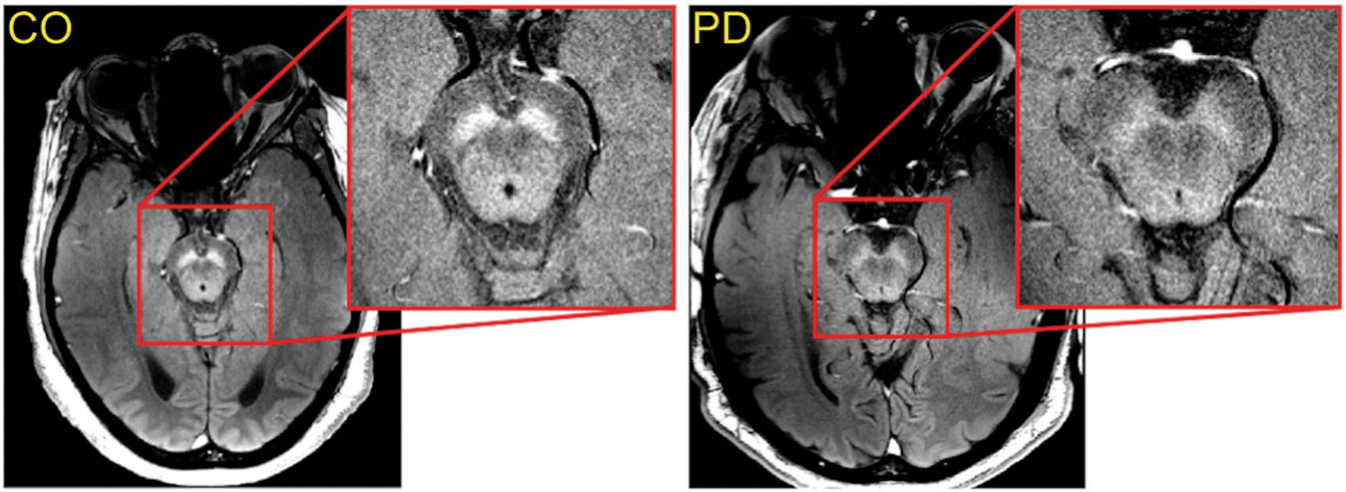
A comparison of SNc CNR in a control subject (labeled CO; left) and a PD patient (labeled PD; right). Both images were acquired using a NM-MRI sequence with an explicit MT preparation pulse^114^ and CNR was calculated using a standardized reference region placed in the cerebral peduncle.^121^ In both images, the red box shows a zoomed in view of the SNc. There is a 25% reduction in SNc CNR of the PD patient compared to SNc CNR of the control subject, indicating a loss of NM pigment in SNc of the PD patient. Identical windowing parameters were used to display both images
